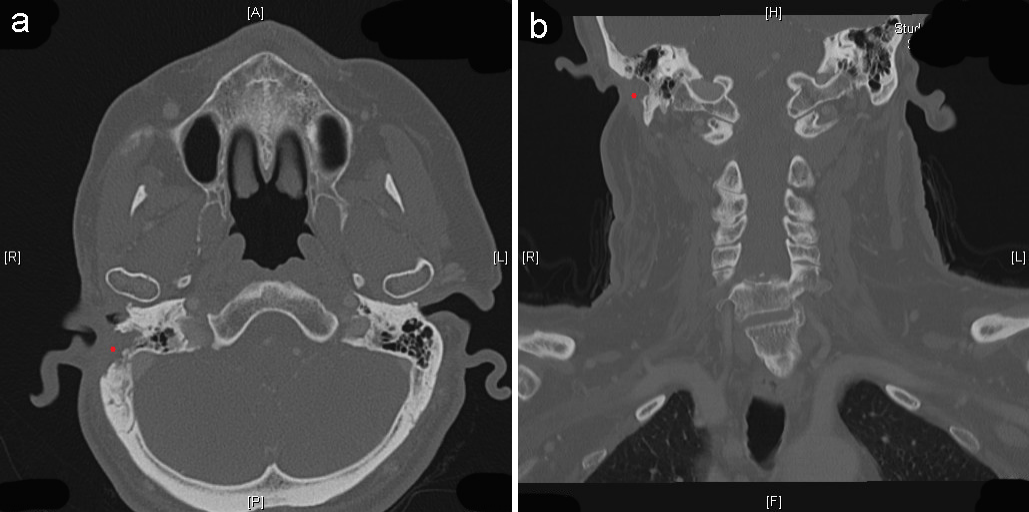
a: Follow-up imaging – axial computerized tomography (CT) section. Red asterisk indicating the partially resected right mastoid process. b: Follow-up imaging – coronal CT section. Red asterisk indicating the partially resected right mastoid process.
